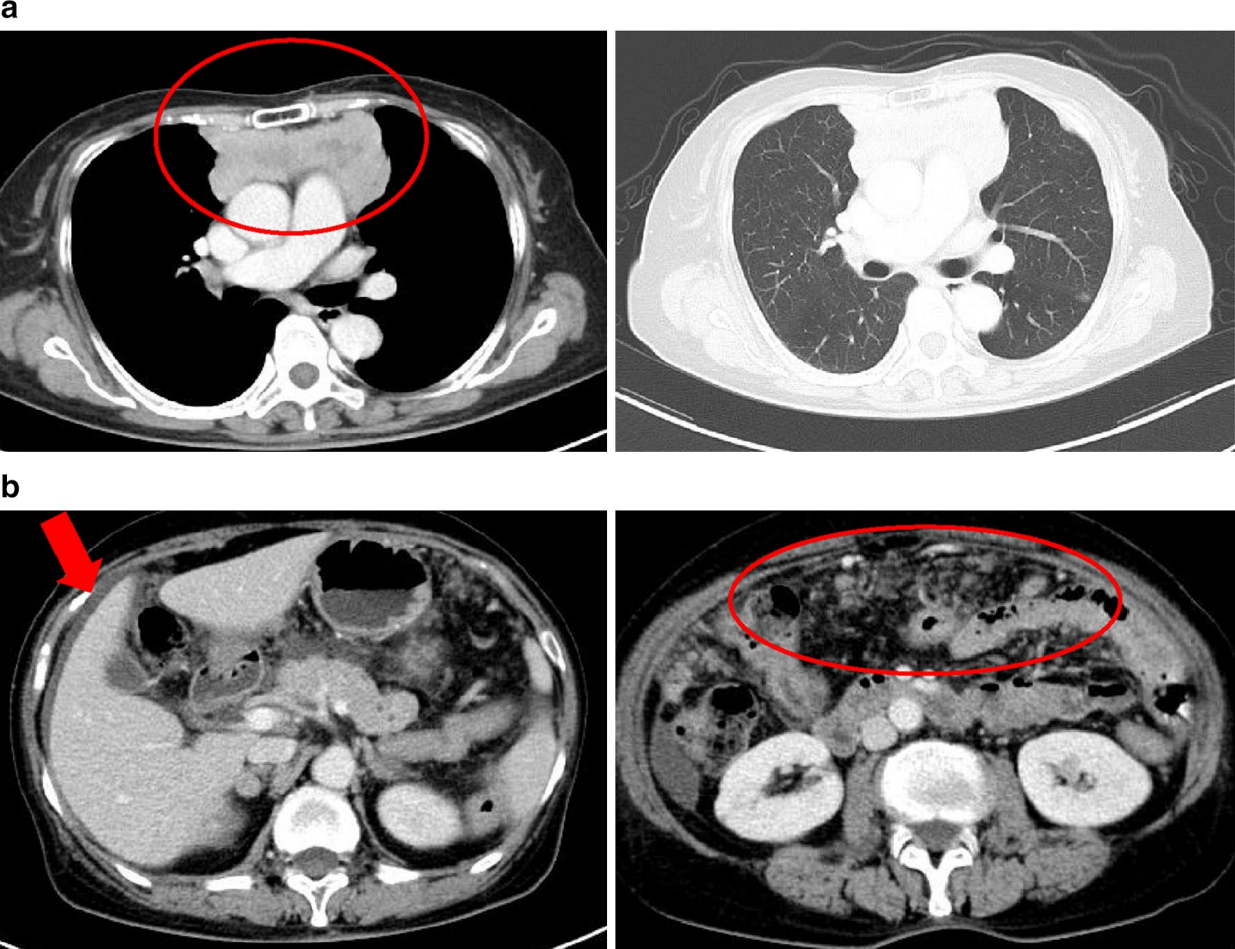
a Chest computed tomography showed an increase in the size of the small cell carcinoma tumor arising from the thymus (*red circle*). b Abdominal computed tomography showed mild ascites (*red arrow*) and prominent irregular granular shadows of the greater omentum and the mesentery (*red circle*).
